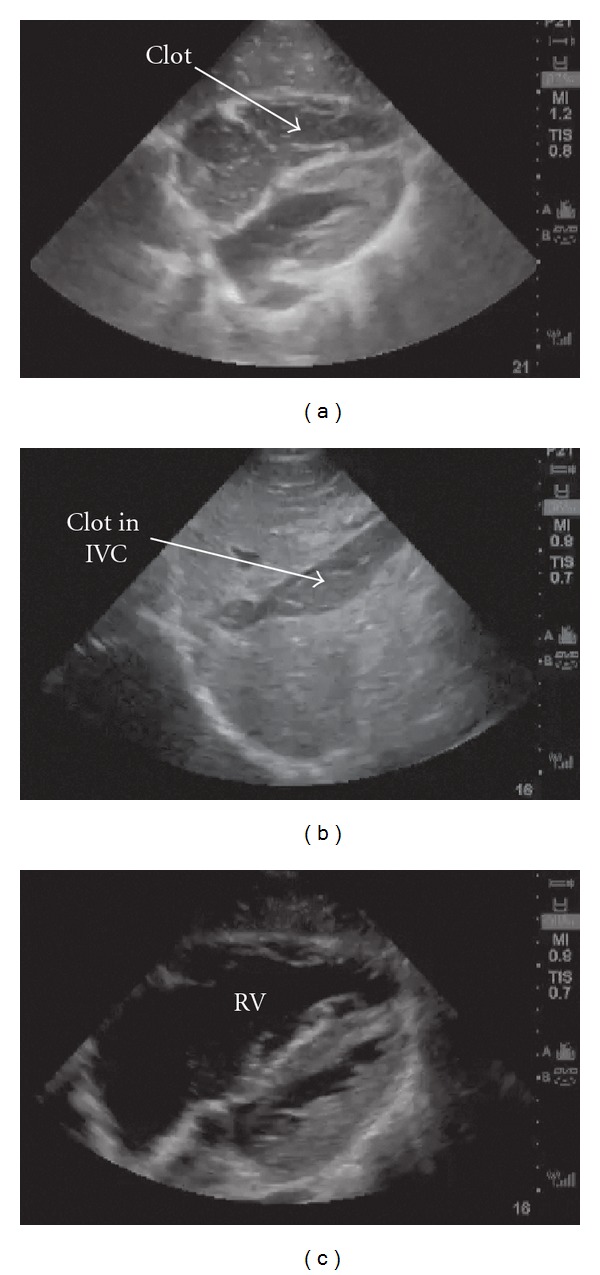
Initial bedside ultrasound demonstrated thrombus in the RV (a) and the IVC (b). A repeat ultrasound demonstrated acute RV enlargement (c).
